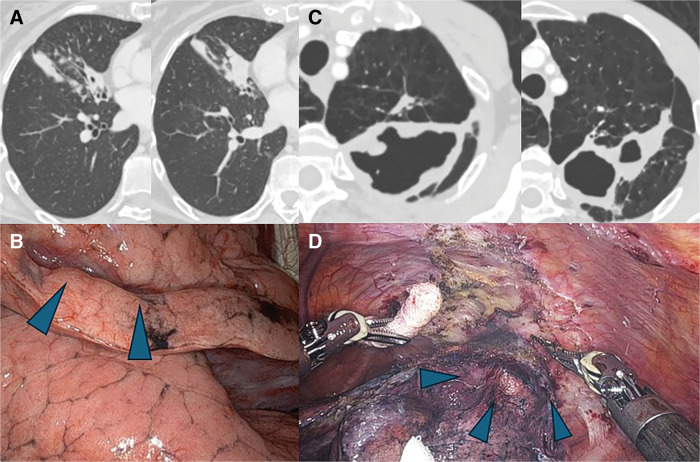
Findings of chest CT and intraoperative findings. (A) Chest CT revealed several nodules and bronchiectasis in the right middle lobe in Case1. (B) There were no adhesions, and the lesion corresponding to the suspected bleeding source was revealed with pleural indentation and neovascularization on the pleural surface overlying the lesion in Case 1 (arrowheads). (C) Chest CT revealed a cavity in the left upper lobe in Case 2. (D) There were ridge adhesions between the parietal pleura and aorta. The blue arrowheads indicate the cavity lesion in Case 2.
